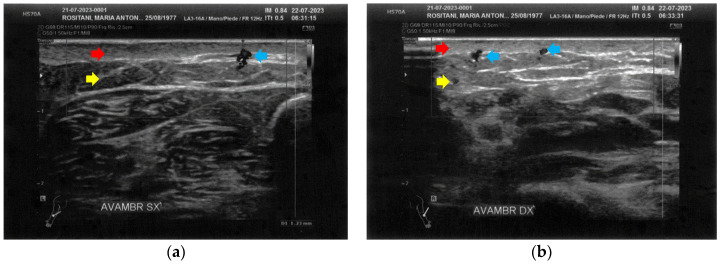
Ultrasonography of the forearms at T1. (a) A significant reduction of the cicatricial fibrosis (red arrow) is evident on the left forearm. A restructuring of all layers is also appreciable (yellow arrow). Moreover, some superficial vascular elements (blue arrow) are present; (b) The right forearm presents the same ameliorations described for the left forearm.
